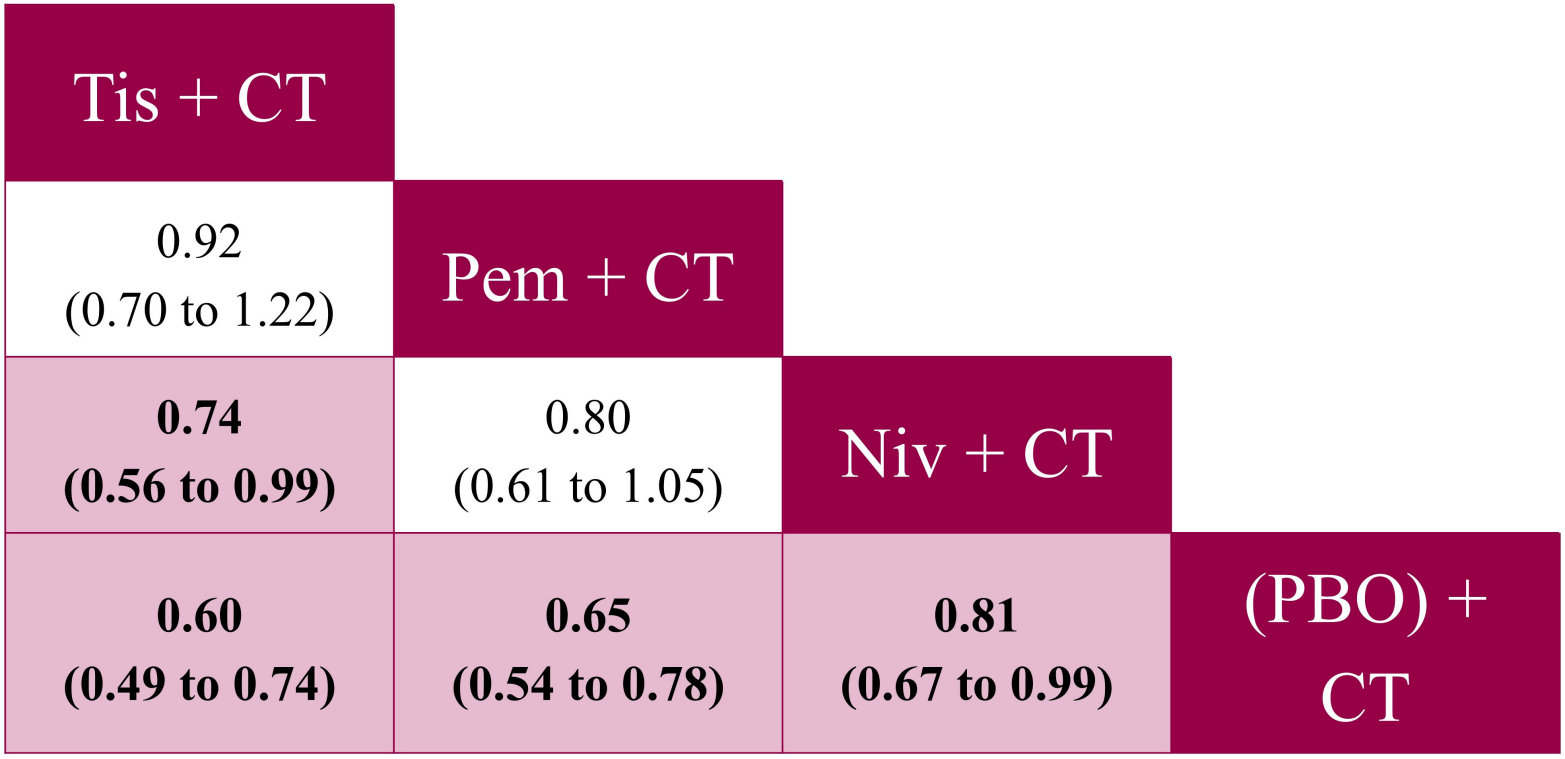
Pairwise comparisons from the fixed effects NMA for PFS (reported as HR [95% CrI]) – ITT population analysis. CrI, credible interval; CT, chemotherapy; HR, hazard ratio; ITT, intent-to-treat; niv, nivolumab; NMA, network meta-analysis; PBO, placebo; pem, pembrolizumab; PFS, progression-free survival; tis, tislelizumab.
